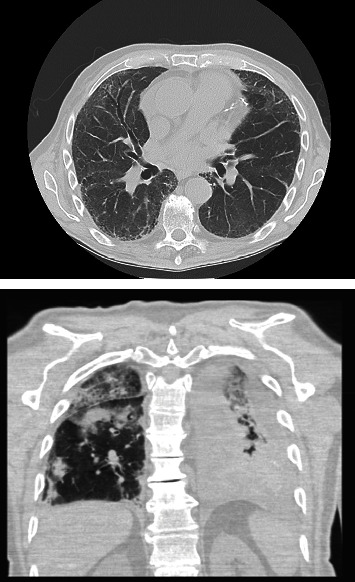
Computed tomography scan of the chest demonstrated multiple infiltrates and ground glass opacities.
